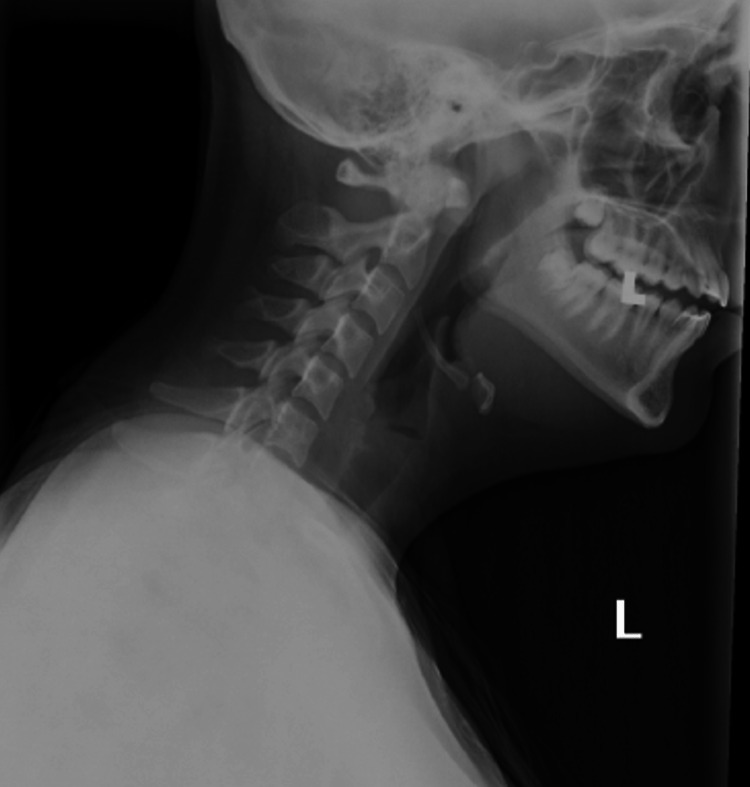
Cervical spine radiograph (lateral view).
